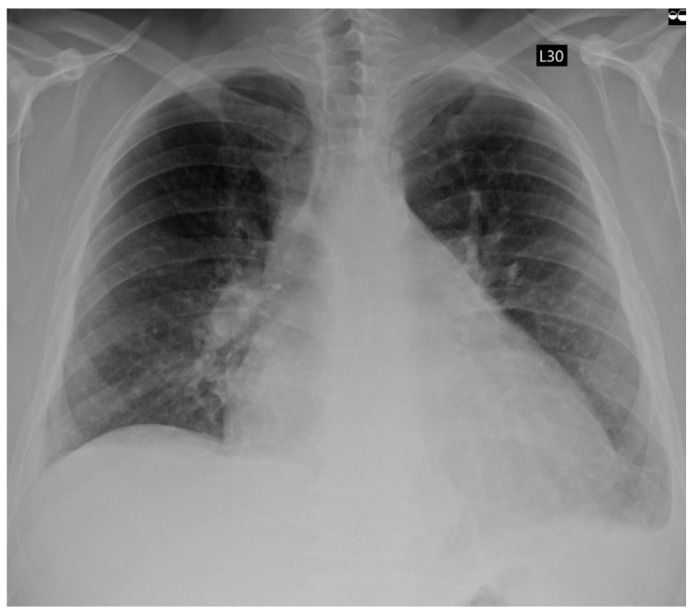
Chest X-Ray showing fluid congestion in admission.
